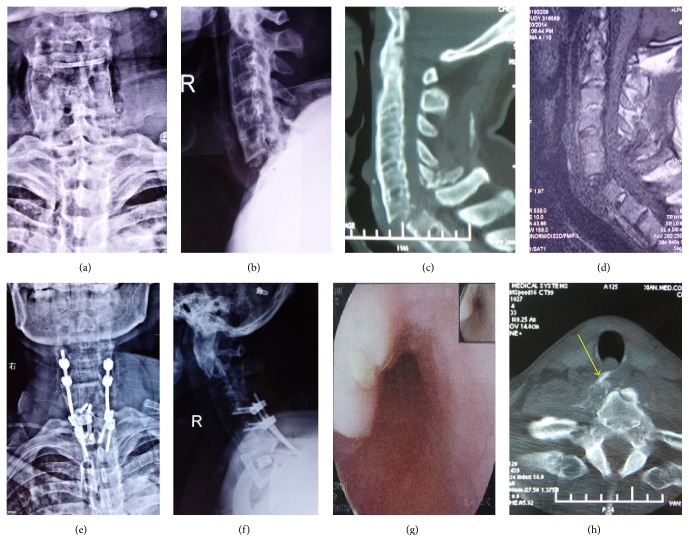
A 53-year-old male was admitted due to sensory-motor disorders of the 4 extremities caused by car accident injuries. (a, b) Anteroposterior and lateral views of an x-ray showing a C6-C7 fracture and dislocation. (c) Sagittal view showing the obviously displaced fracture and dislocation of C6-C7. (d) MRI showing a long T1 signal in the anterior cervical spine. (e, f) Anteroposterior and lateral views showing good position of internal fixation and good reduction. (g) Oesophagoscopy showing a suspicious finding of the tracheoesophageal fistula. (h) Compression of the soft tissue anterior to C6 caused by vertebral fracture fragments (yellow arrow).
